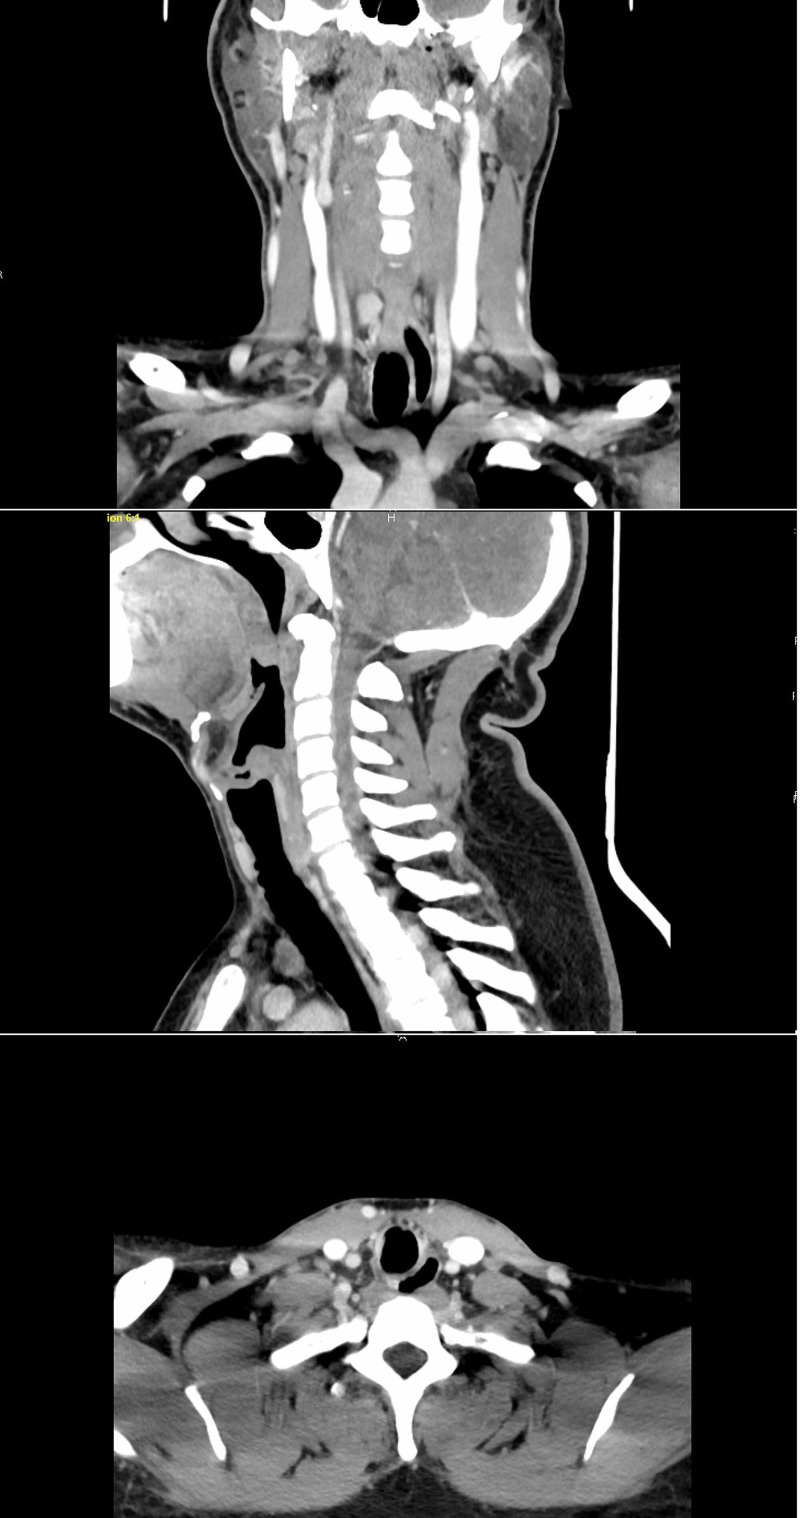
Follow up soft tissue neck CT scan confirming complete expulsion of the obstructive lesion
